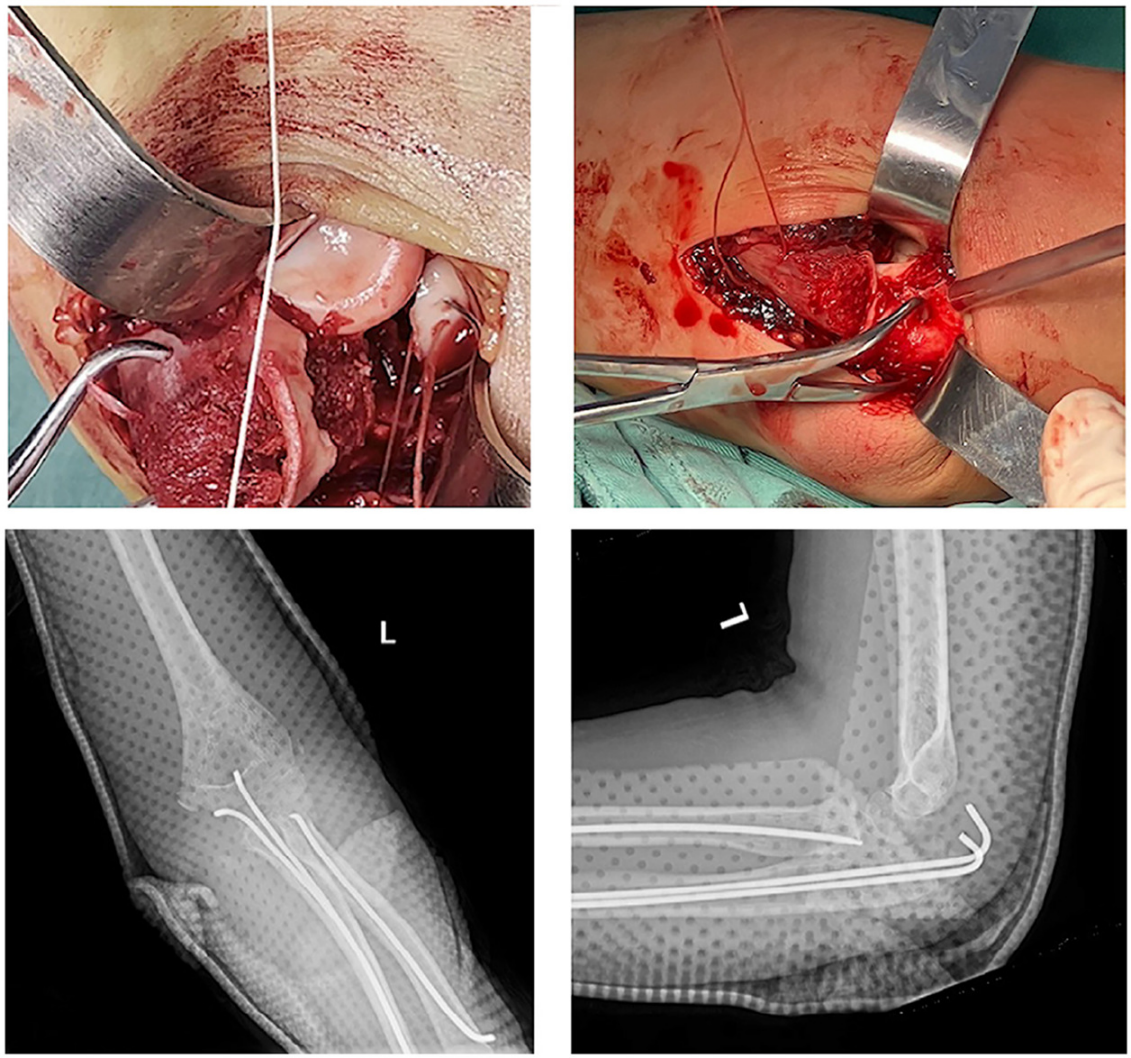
The coronoid process of the ulna fixed using the suture lasso technique and postoperative x-rays of the elbow joint.
